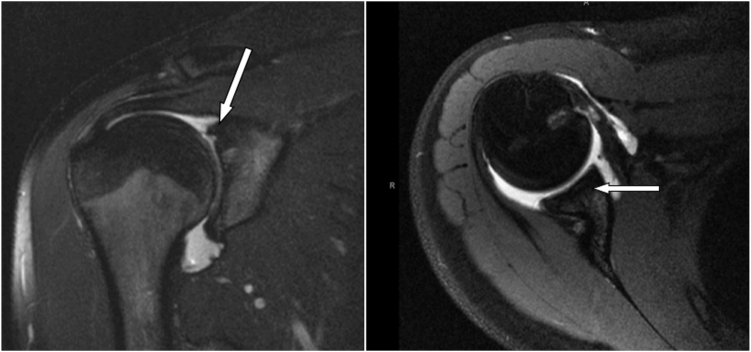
One-year postoperative coronal (*left*) and axial (*right*) T2-weighted MRI of right shoulder demonstrating healed subscapularis and incorporation of glenoid graft at the anterosuperior margin of the glenoid. *MRI*, magnetic resonance image.
